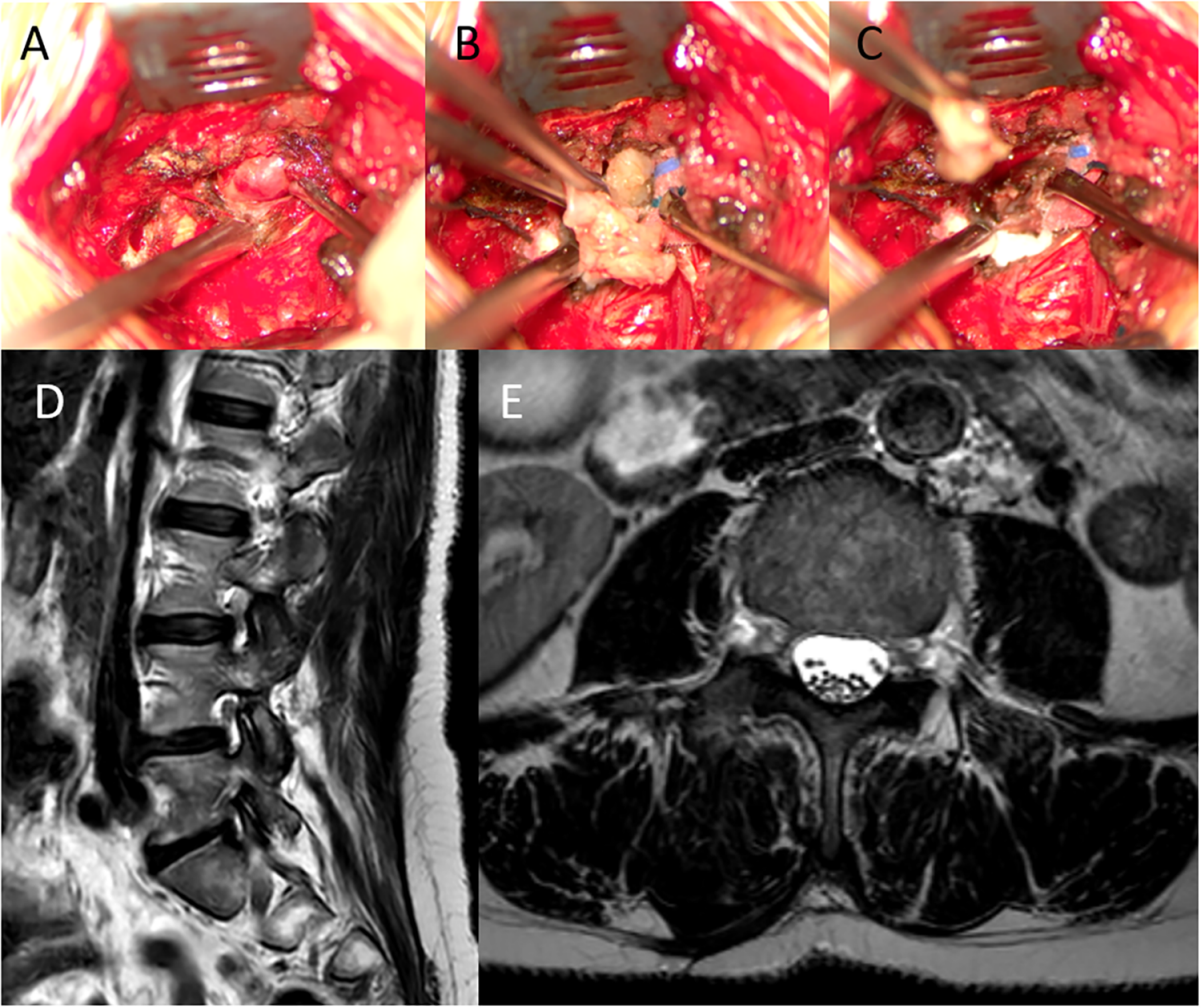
Intraoperative view (A, B, C) and follow-up MRI (D, E). Tumor was approached via O-Arm neuronavigated Wiltse approach. (A) Exposure of the tumor. (B) Opening of the capsule. (C) Schwannoma removal. It can be appreciated how skin incision and soft tissue dissection in perfectly centered over the lesion. (D) Sagittal and (E) axial T2 WI 6-months follow-up spine MRI showing the gross total tumor resection.
